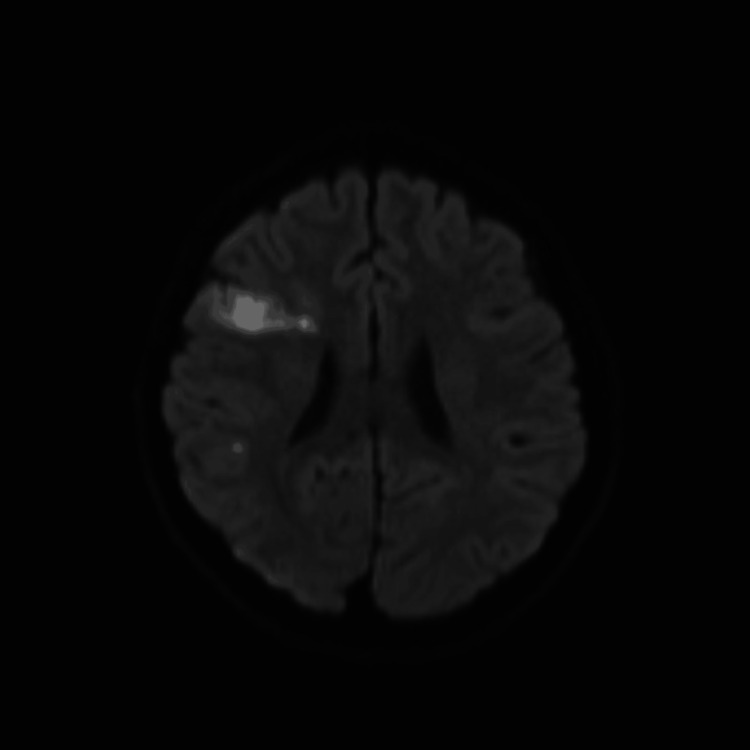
MRI, Axial DWI image showing diffusion restriction in the right frontal lobe in favor of ischemia.
